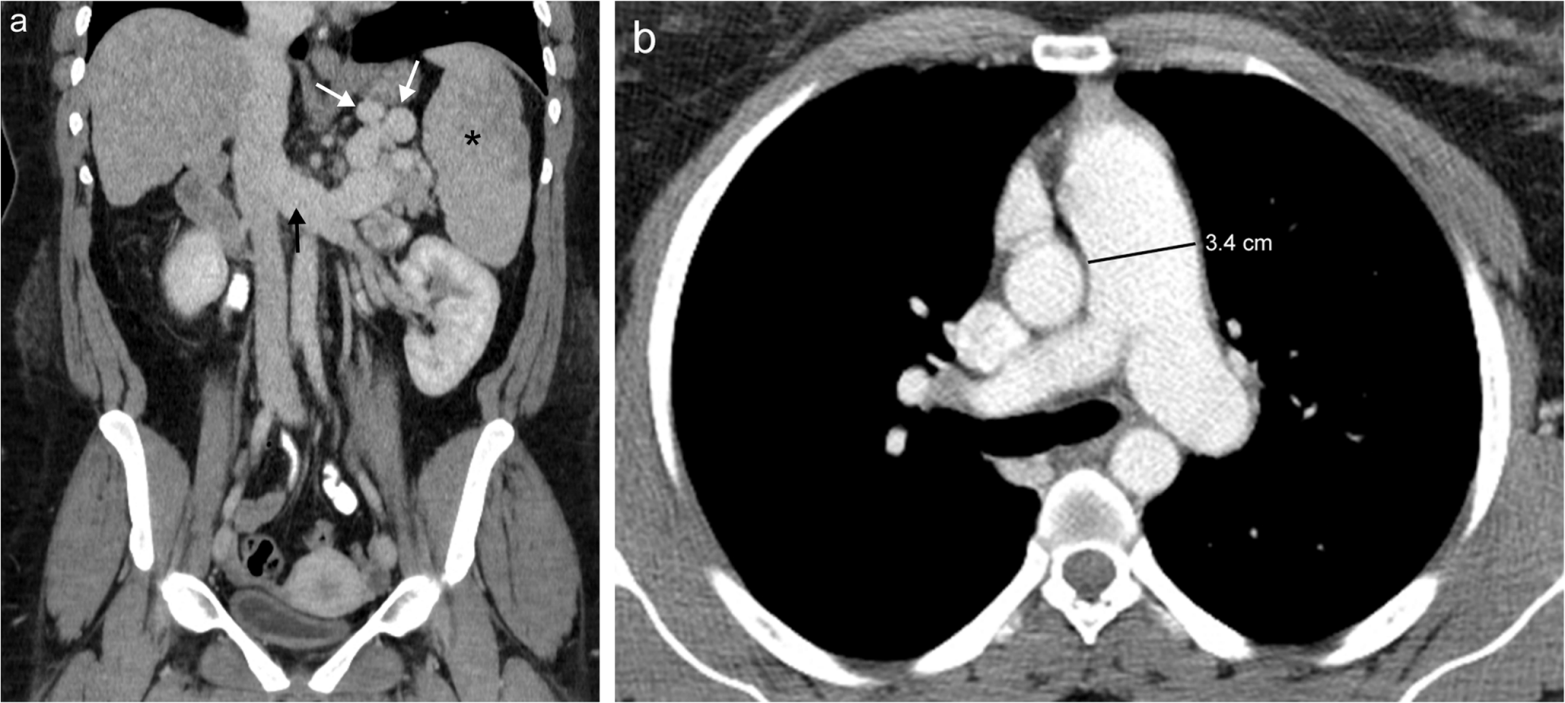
Pulmonary hypertension in a patient with liver disease. a Coronal contrast-enhanced CT image shows sequela of portal hypertension secondary to cirrhotic liver morphology with splenomegaly (*), enlarged splenorenal collateral vessels (*white arrows*) and enlarged left renal vein (*black arrow*). b Axial contrast-enhanced CT image shows dilatation of the pulmonary arteries with the main pulmonary artery measuring up to 3.4 cm in maximal diameter
